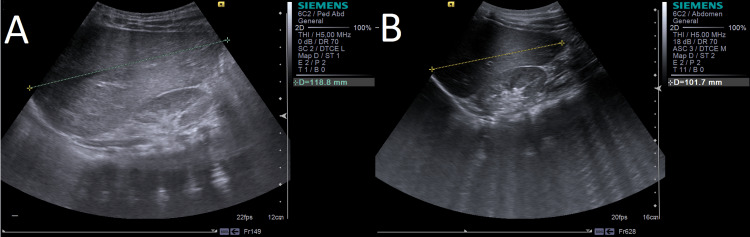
Liver ultrasound at 28 months (A) and 44 months (B). (A) Hepatomegaly at 28 months, with right lobe sizing 118.8 mm. (B) Right lobe with 101.7 mm, showing liver size reduction at 44 months.
